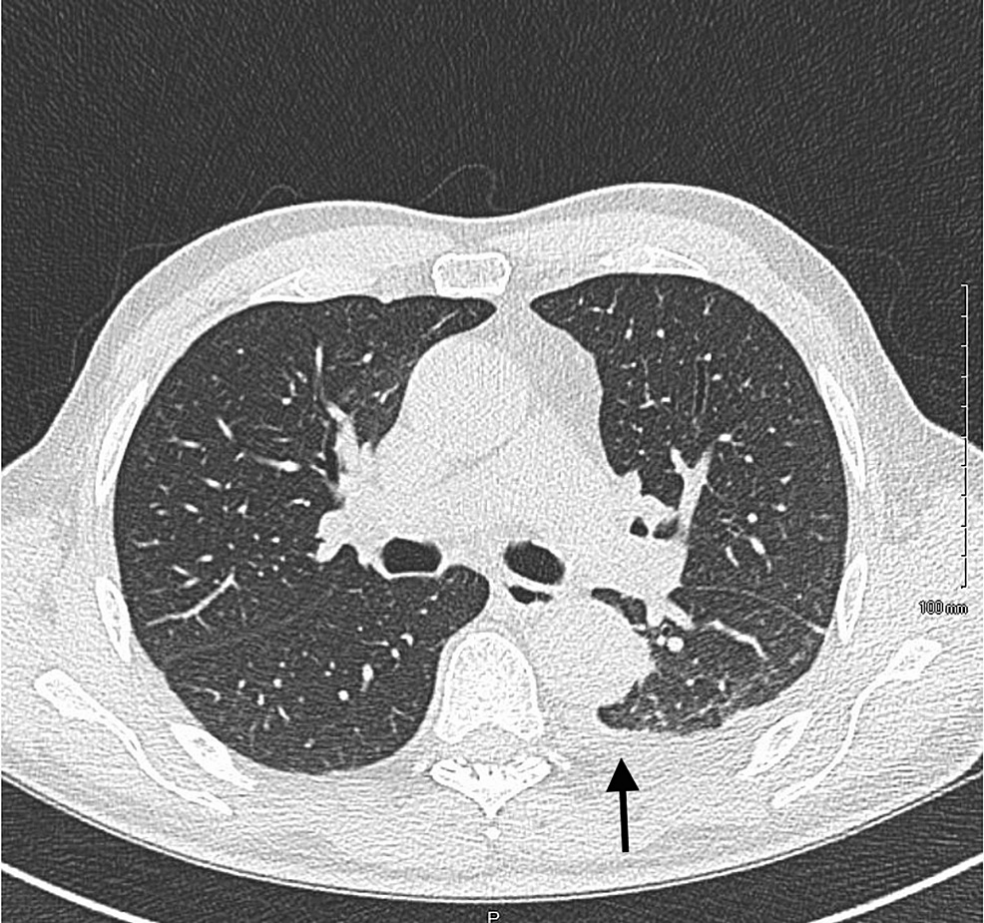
Chest Computed Tomography (CT) Scan A chest computed tomography (CT) showing a left-sided posterior fluid collection (arrow).
